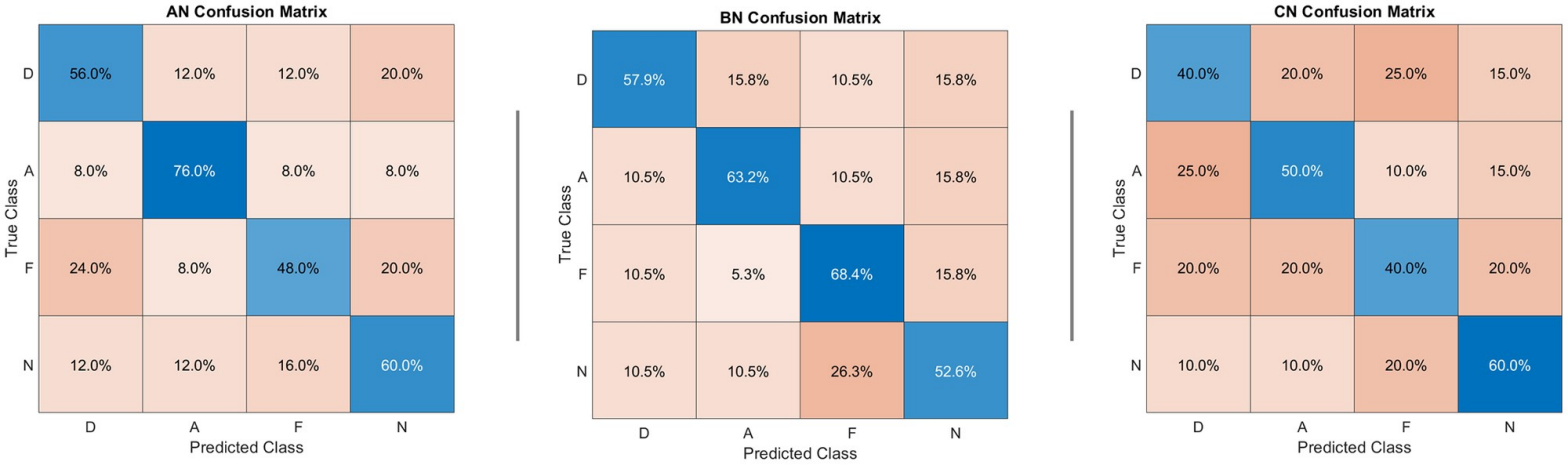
Confusion matrix. AN = Anorexia Nervosa, BN = Bulimia Nervosa, CN = Normal Controls. D = disgust, A = anger, F = fear, N = neutral. Predicted (by the MVPA) vs. real emotion for each stimulus presented during the functional MRI task. No pairwise comparison between matrices is significant.
